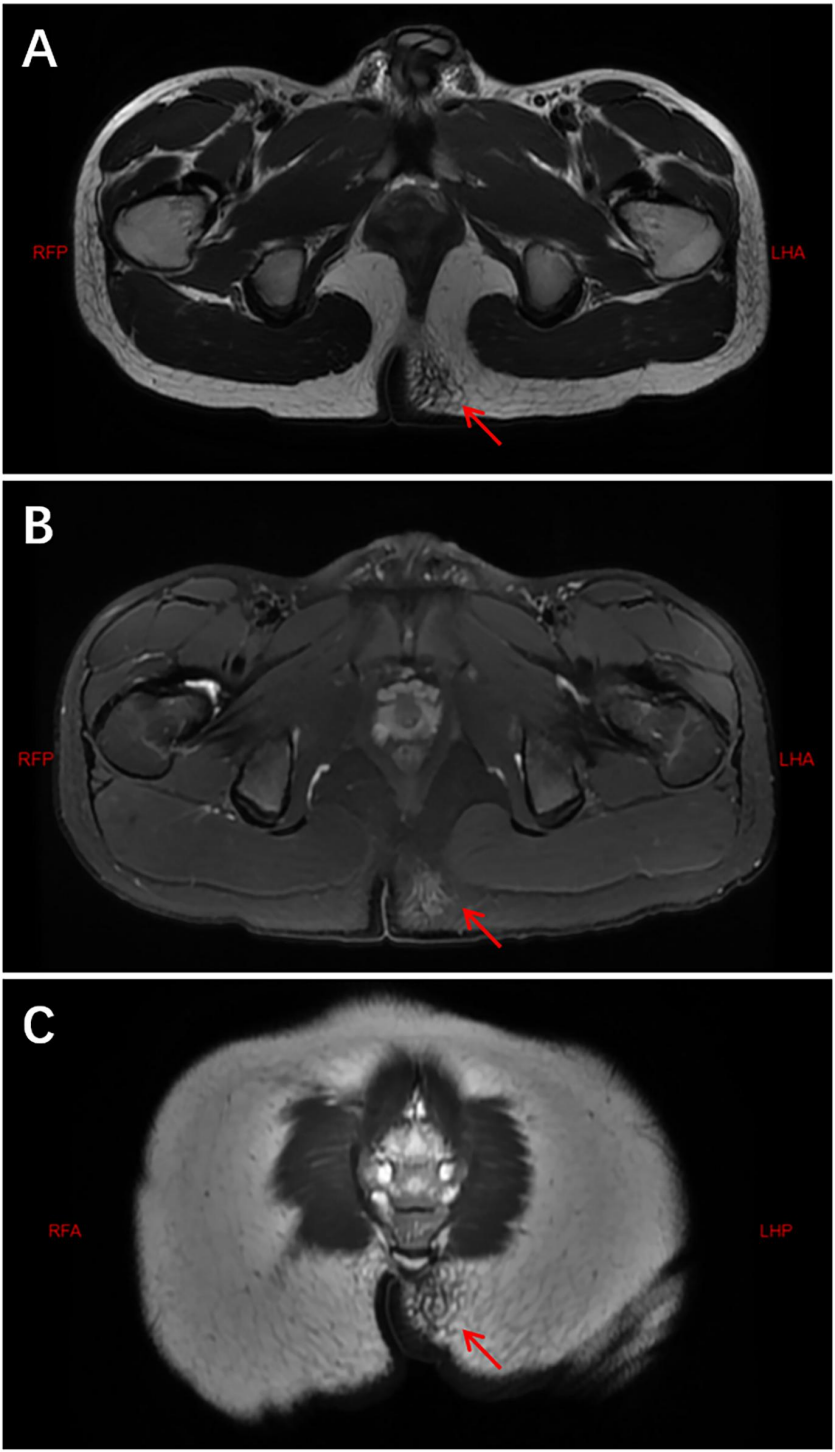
MRI findings of the left gluteal lesion. (A) T1-weighted image reveals a slightly hypointense mass within the subcutaneous fat. (B) The mass appears mildly hyperintense on T2-weighted imaging. (C) Fat-suppressed sequence demonstrates a hyperintense lesion with ill-defined margins, measuring approximately 3.0 cm × 2.3 cm × 1.9 cm.
